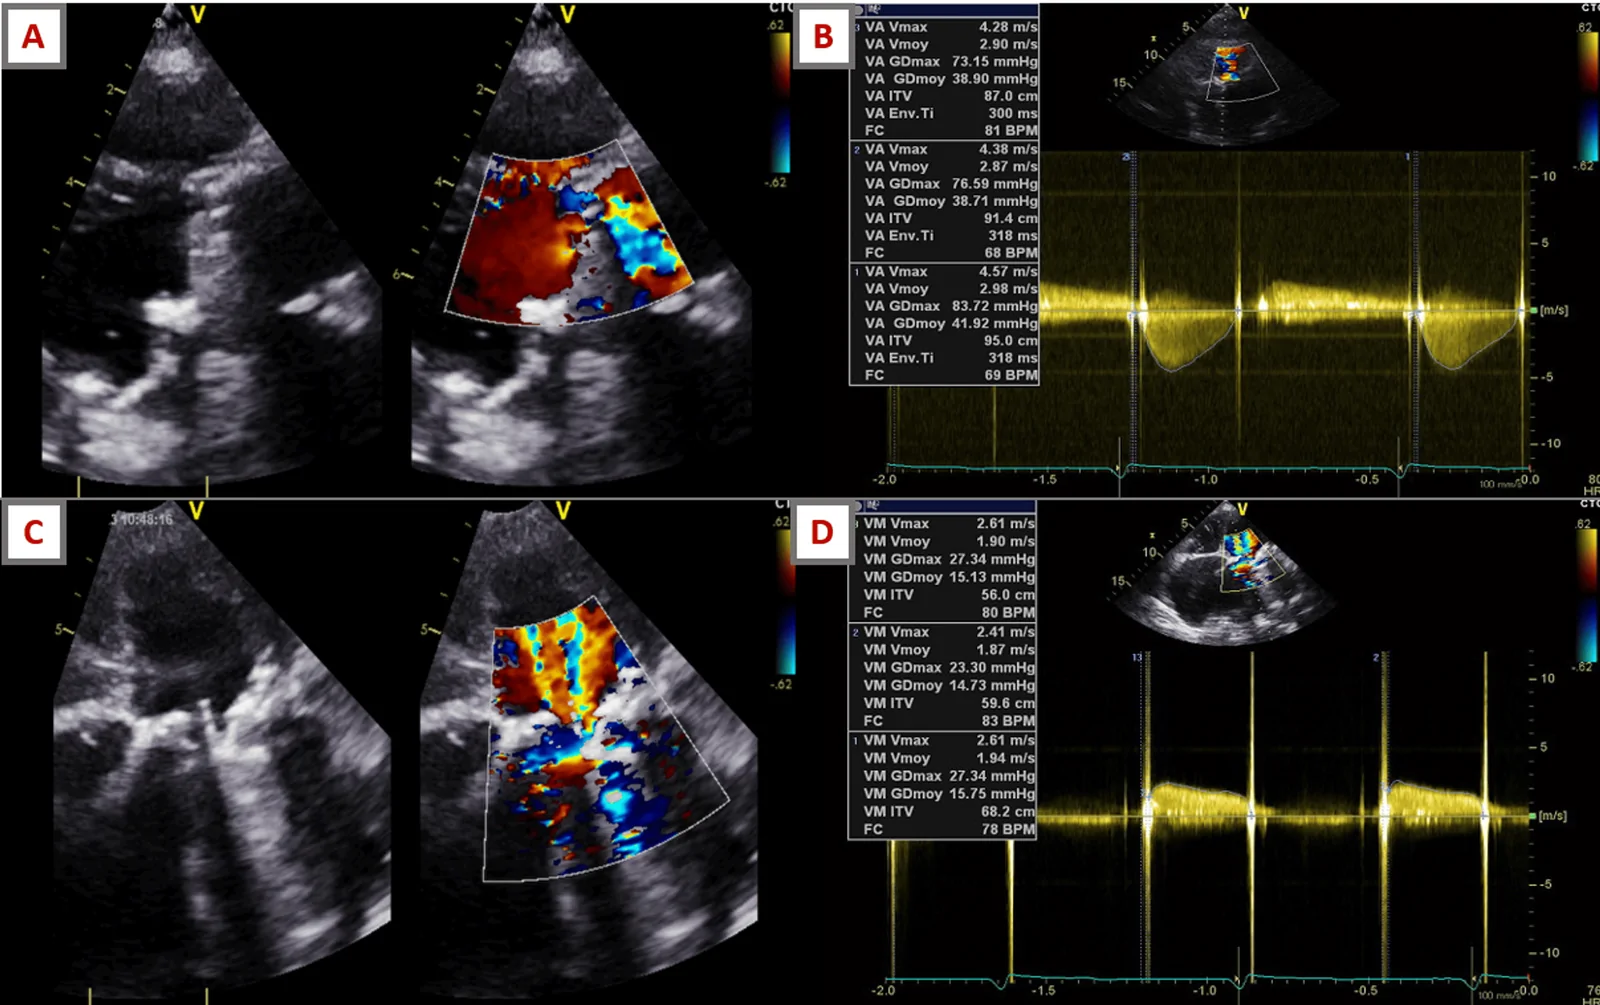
TTE images showing markedly elevated transprosthetic gradients across both the mitral and aortic prostheses. (A) Color Doppler imaging demonstrating flow acceleration with aliasing at the level of the aortic prosthesis. (B) Continuous-wave Doppler across the aortic prosthetic valve showing a markedly elevated mean gradient of 40 mmHg averaged over multiple cardiac cycles. (C) Color Doppler imaging demonstrating significant flow acceleration with aliasing at the level of the mitral prosthesis. (D) Continuous-wave Doppler across the mitral prosthetic valve showing a markedly elevated mean gradient of 15.2 mmHg averaged over multiple cardiac cycles. TTE, transthoracic echocardiography
